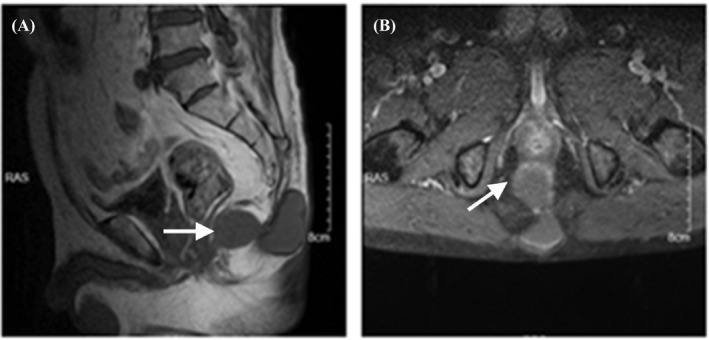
High‐resolution computed tomography (HRCT) of the sacrococcygeal vertebrae showed oval cystic lesions involving in subcutaneous tissue and pelvic cavity. Figure A and Figure B represented the location and size of the abscess under the lateral position and frontal position, respectively. There was an oval cystic lesion near the fifth cone of sacrum and subcutaneous tissue, respectively, and the two lesions were connected. The size of the larger abscess was about 50 × 30 mm, and the size of the smaller abscess was about 40 × 30 mm. White arrow indicated the abscesses
